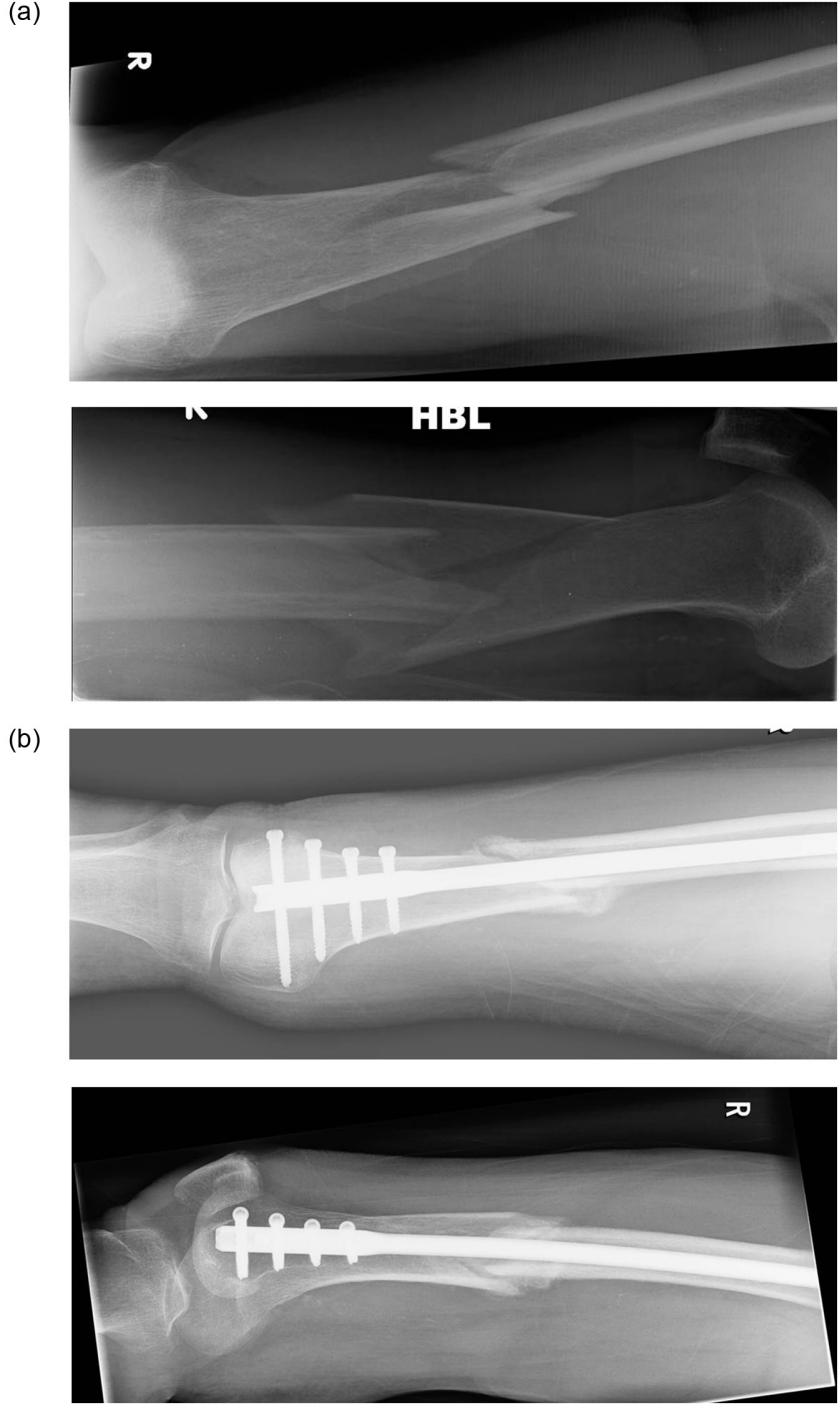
(a) Pre- and (b) post-operative radiographs of distal femur fracture treated with Targon RF nail.
